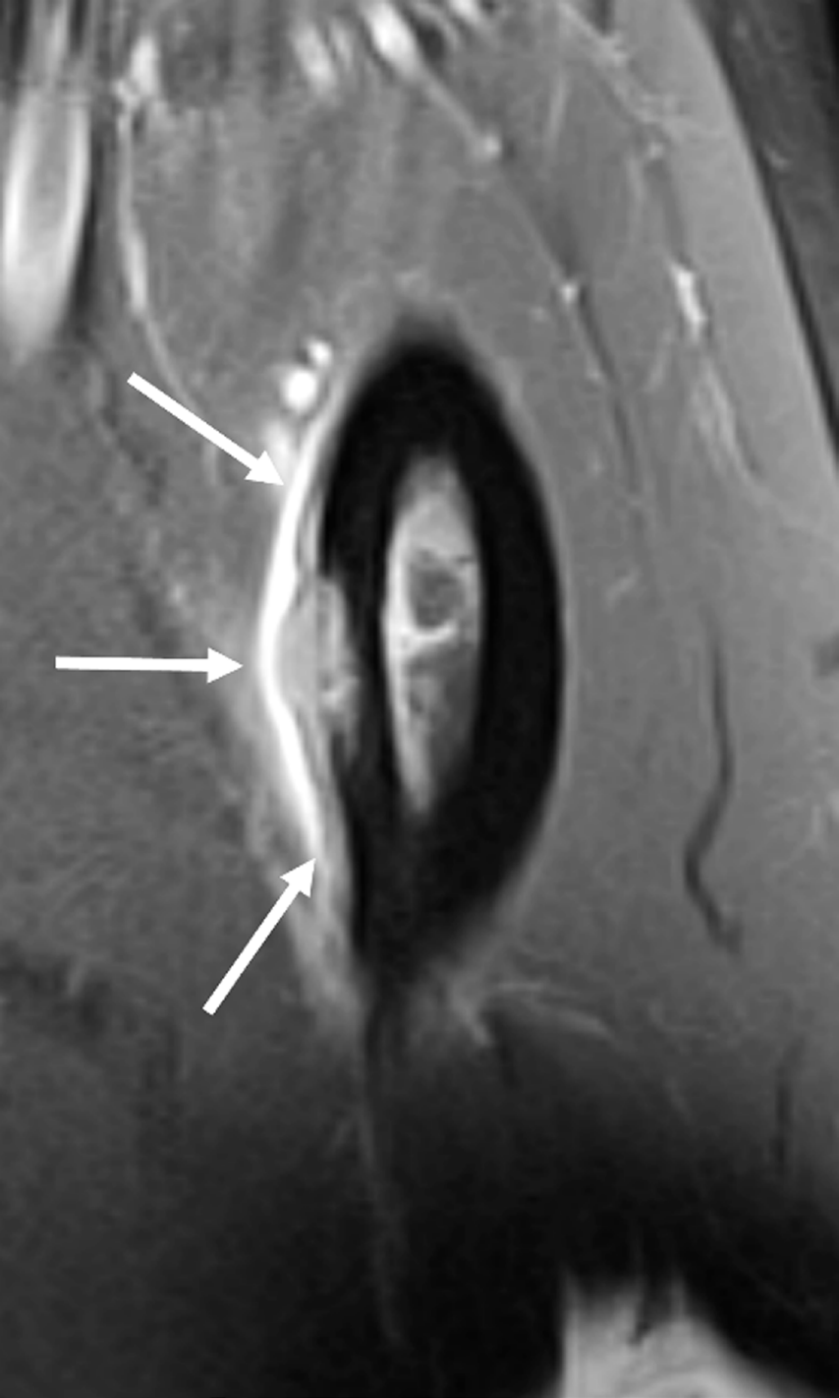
The primary diagnostic consideration was a cortical metastasis, and exploration on a primary tumor was initiated. The patient had a long-term history of smoking and computed tomography (CT) of the chest revealed a primary lung tumor (Figure 3A, arrow) with unilateral hilar lymphadenopathy (Figure 3B, arrow).
